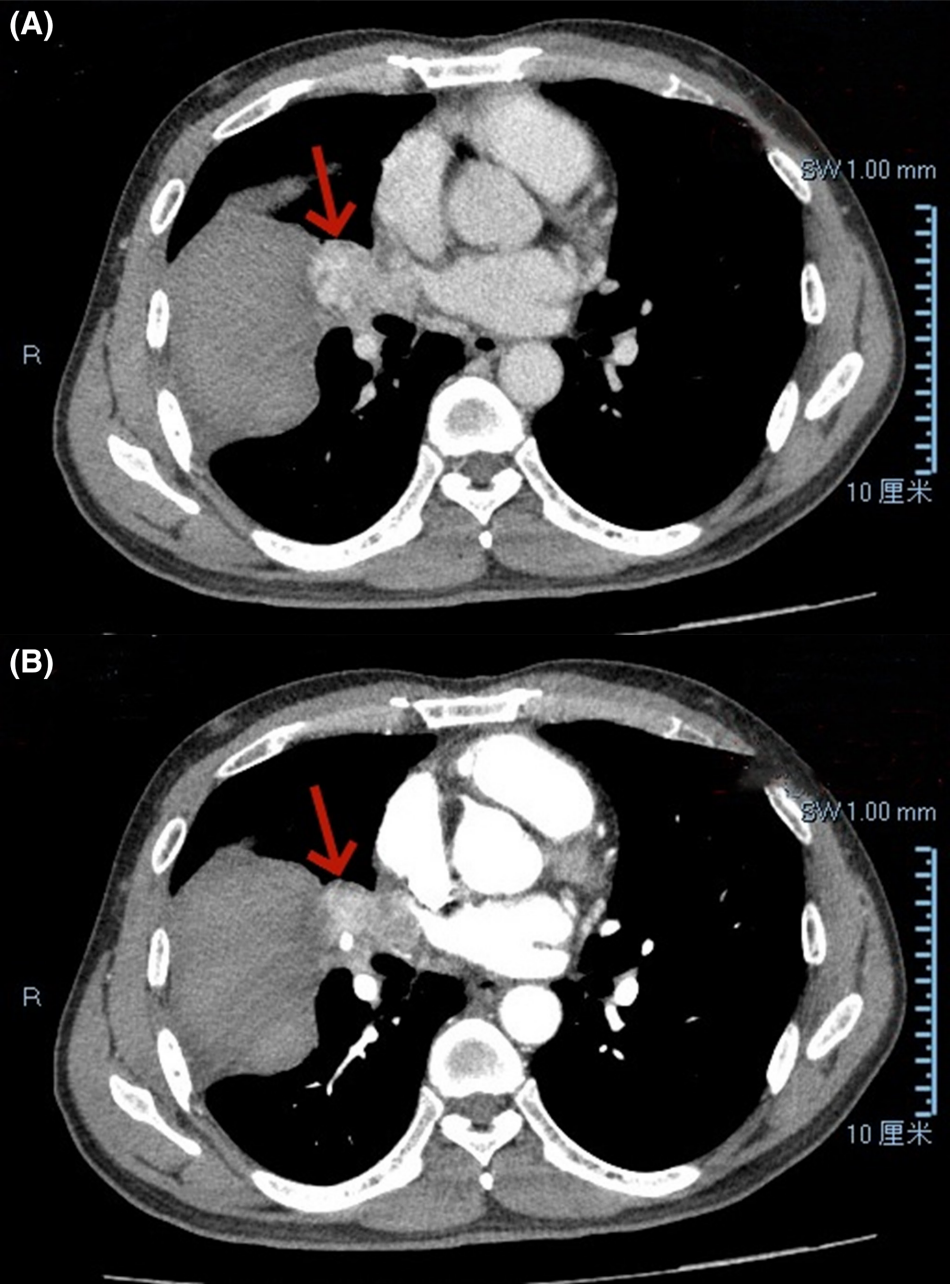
The chest contrast‐enhanced computed tomography (CT) image showed a mass in the right middle lobe of the lung near the hilus and a right horizontal interlobar hematoma. (A) Shows the venous phase appearance of chest enhanced CT; (B) Shows the arterial phase appearance.
